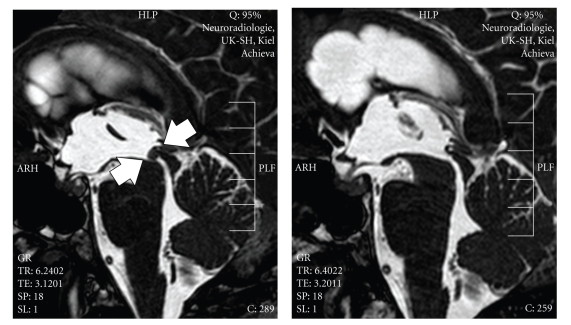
T2-weighted sagittal MRI (left) before and (right) after endoscopic tumor removal with restoration of the aqueduct. The tumor is marked by arrows.
